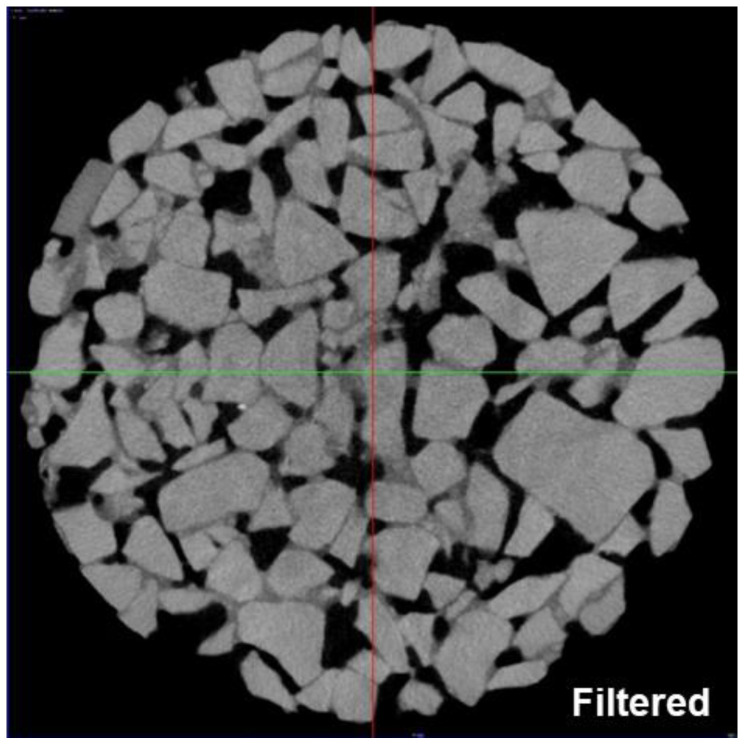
Two-dimensional-image from CT data after image noise reduction. The image corresponds to the same sample location (slice No. 760), which is also shown in Figure 4a before image noise reduction. The sample diameter is 100 mm. Pores = black; aggregates = bright gray; mastic = dark gray.
